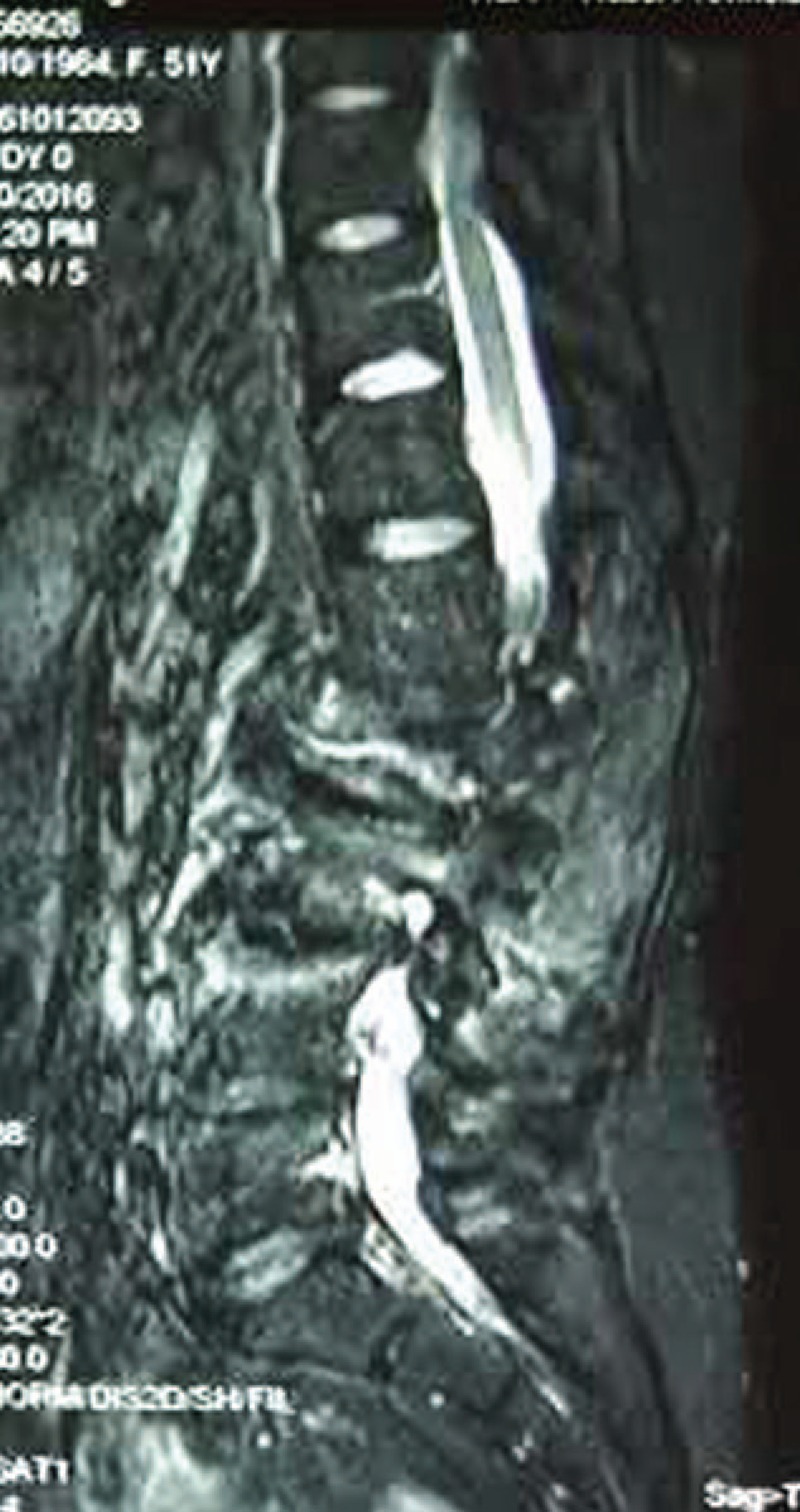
MRI for lumbar showed no neurothlipsis. MRI = magnetic resonance imaging.
